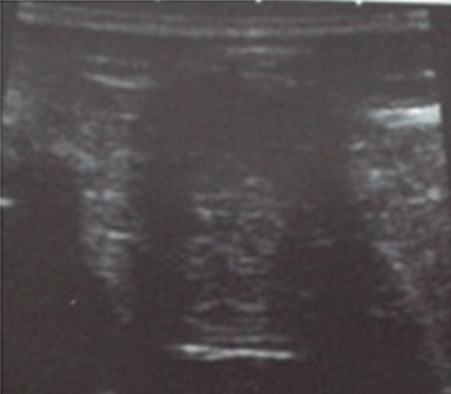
Lipoma in a 40-year-old woman with a palpable lump in the right breast; Ultrasound examination: ultrasonography showed a predominantly hypoechoic lesion with posterior shadowing
